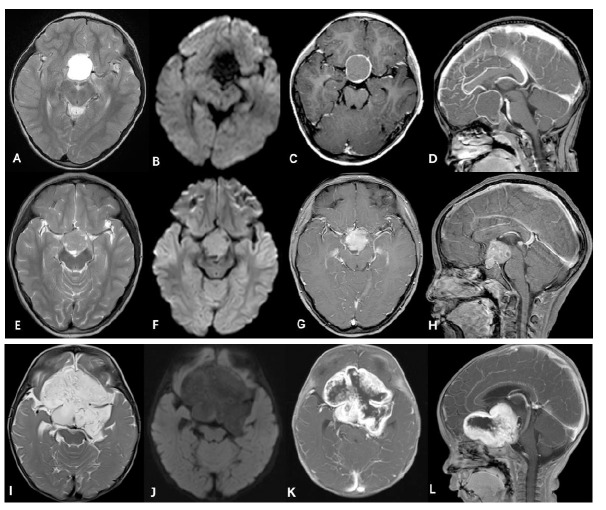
A pathologically confirmed ACP in a 6-year-old girl. It was a cystic lesion, exhibiting hyperintensity on T2WI (A) and no restriction on DWI (B). Following contrast administration, the ACP demonstrated significant ring-like enhancement in the cystic wall (C and D). A pathologically confirmed suprasellar germinoma in an 11-year-old girl. The tumor displayed slight hyperintensity on T2WI (E), typical hyperintensity on DWI (F), and uniform and pronounced enhancement post-contrast (G and H). A pathologically confirmed suprasellar PMA in an 8-month-old boy. It presented as a large complex solid-cystic tumor with hyperintensity on T2WI (I) and no restriction on DWI (J). After contrast (K, L), the tumor showed heterogeneous rim enhancement.
